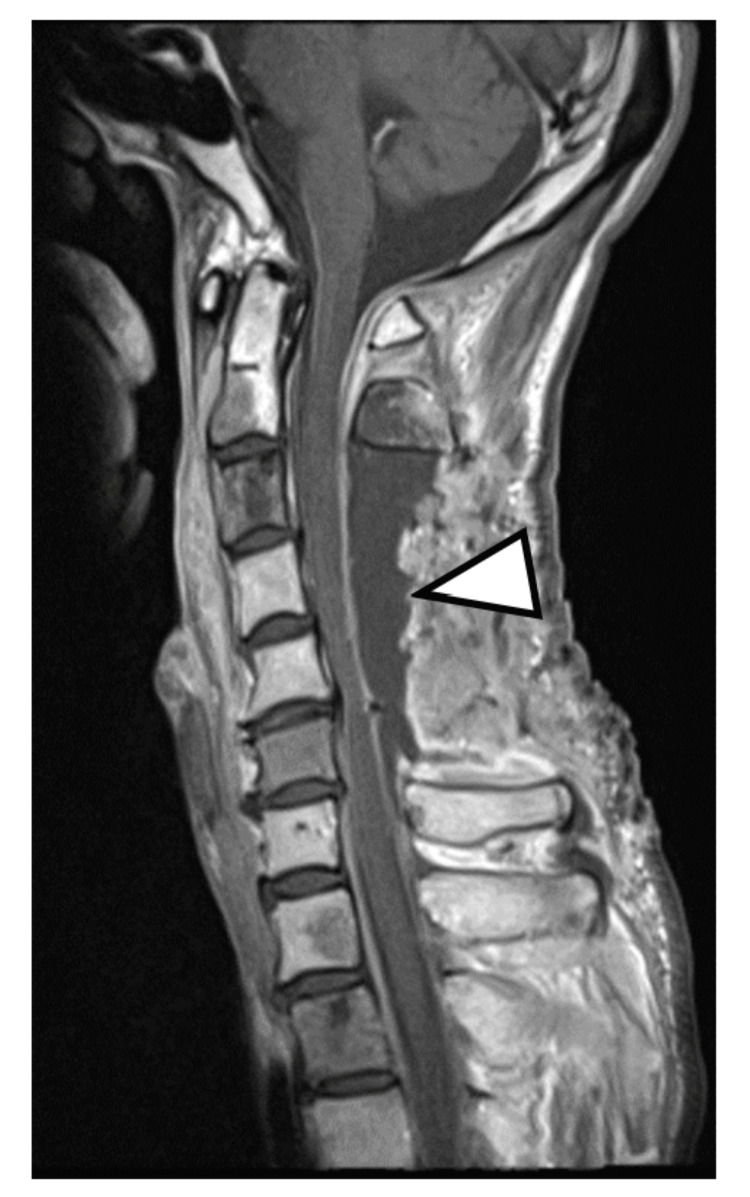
Contrast-enhanced cervical spine MRI (T1-weighted sagittal image) obtained 27 days after reoperation Contrast enhancement of the cervical dura was observed, suggesting the dissemination of cancer cells in the cerebrospinal fluid. No obvious spinal cord compression was noted (arrowhead) MRI: magnetic resonance imaging
